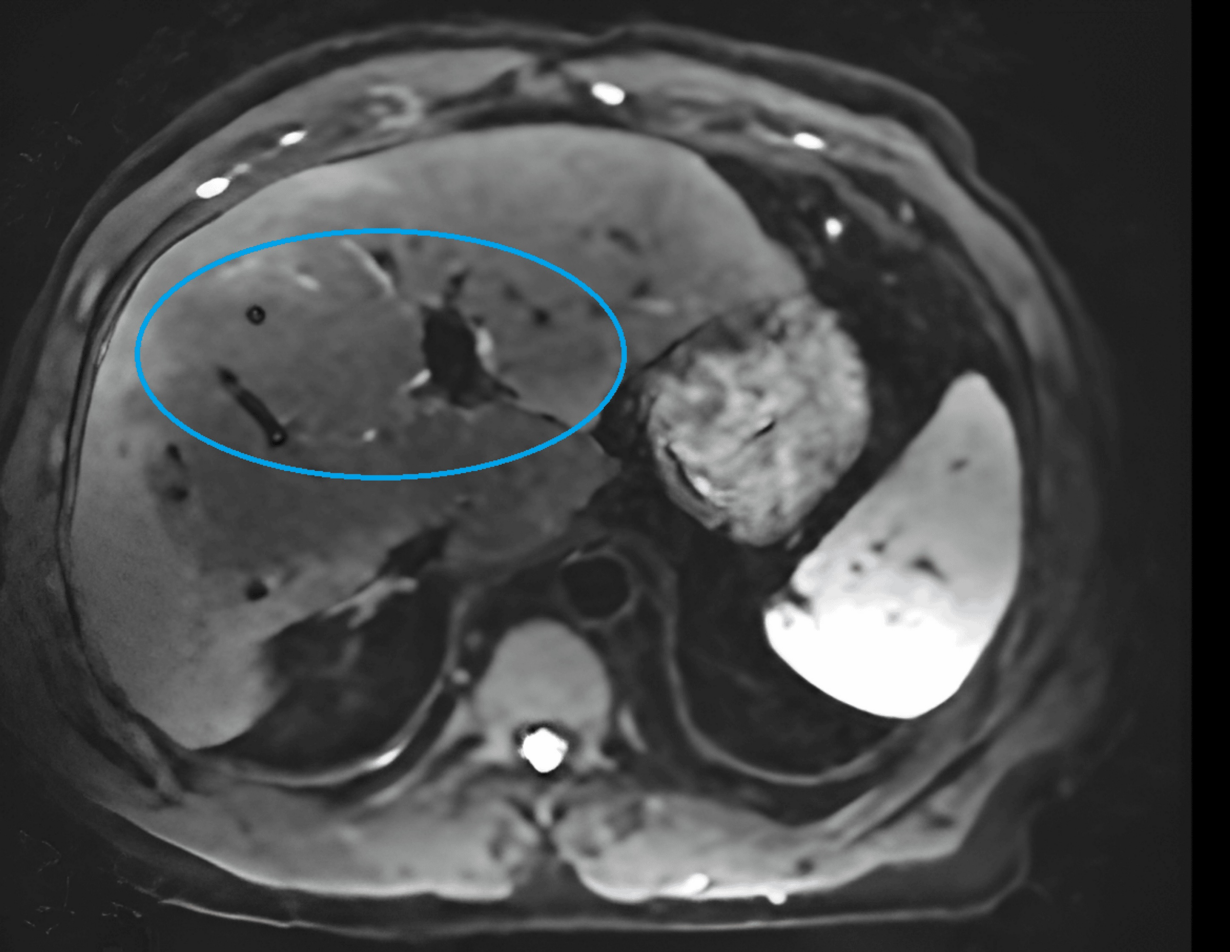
MRI of liver The MRI image demonstrates nodular hepatic contour suggestive of chronic parenchymal disease and peripheral geographic signal changes in the right hepatic lobe with capsular retraction are suggestive of confluent hepatic fibrosis.
